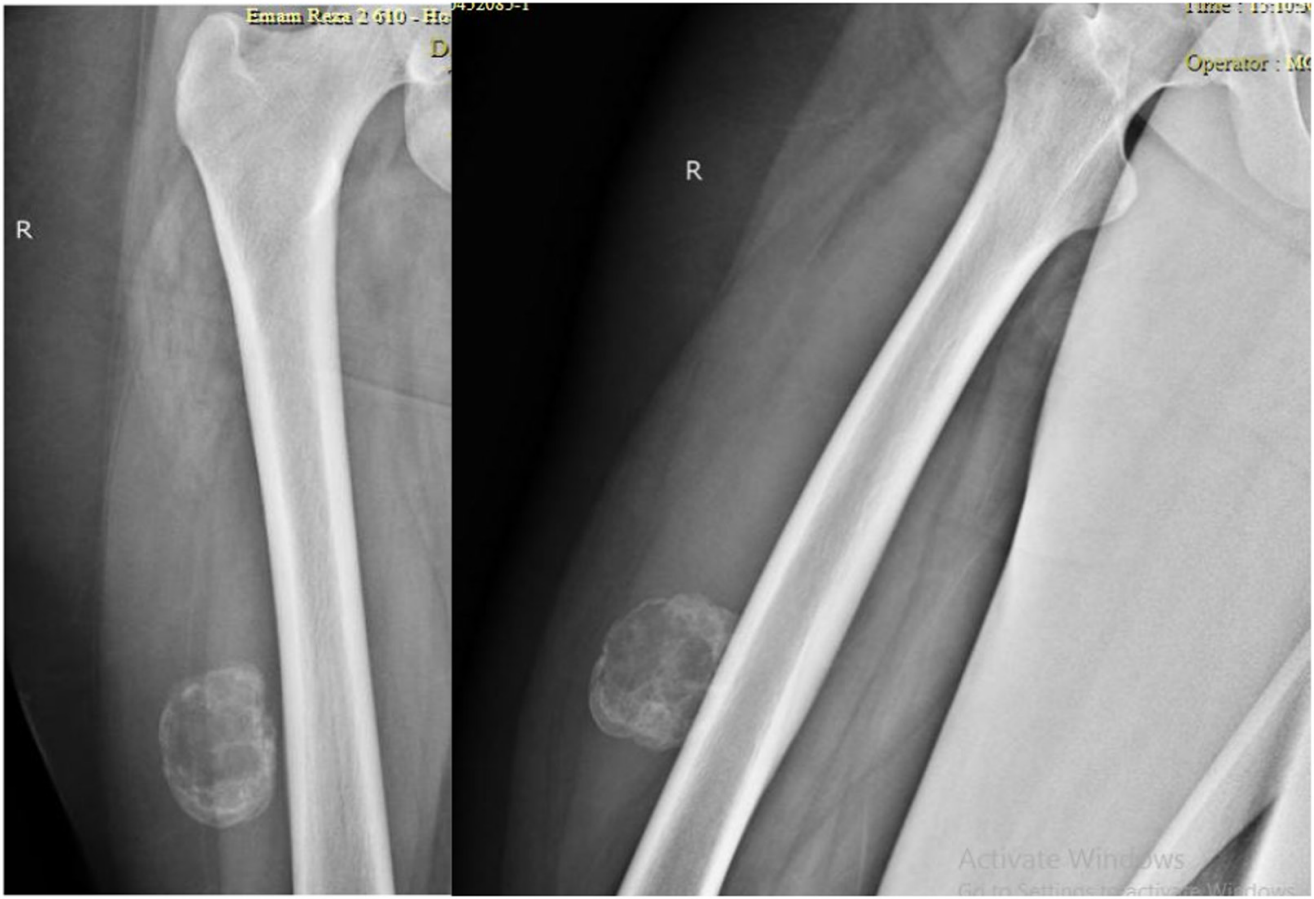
Right lower limb radiographs showing soft tissue mass with well‐defined calcified margins
